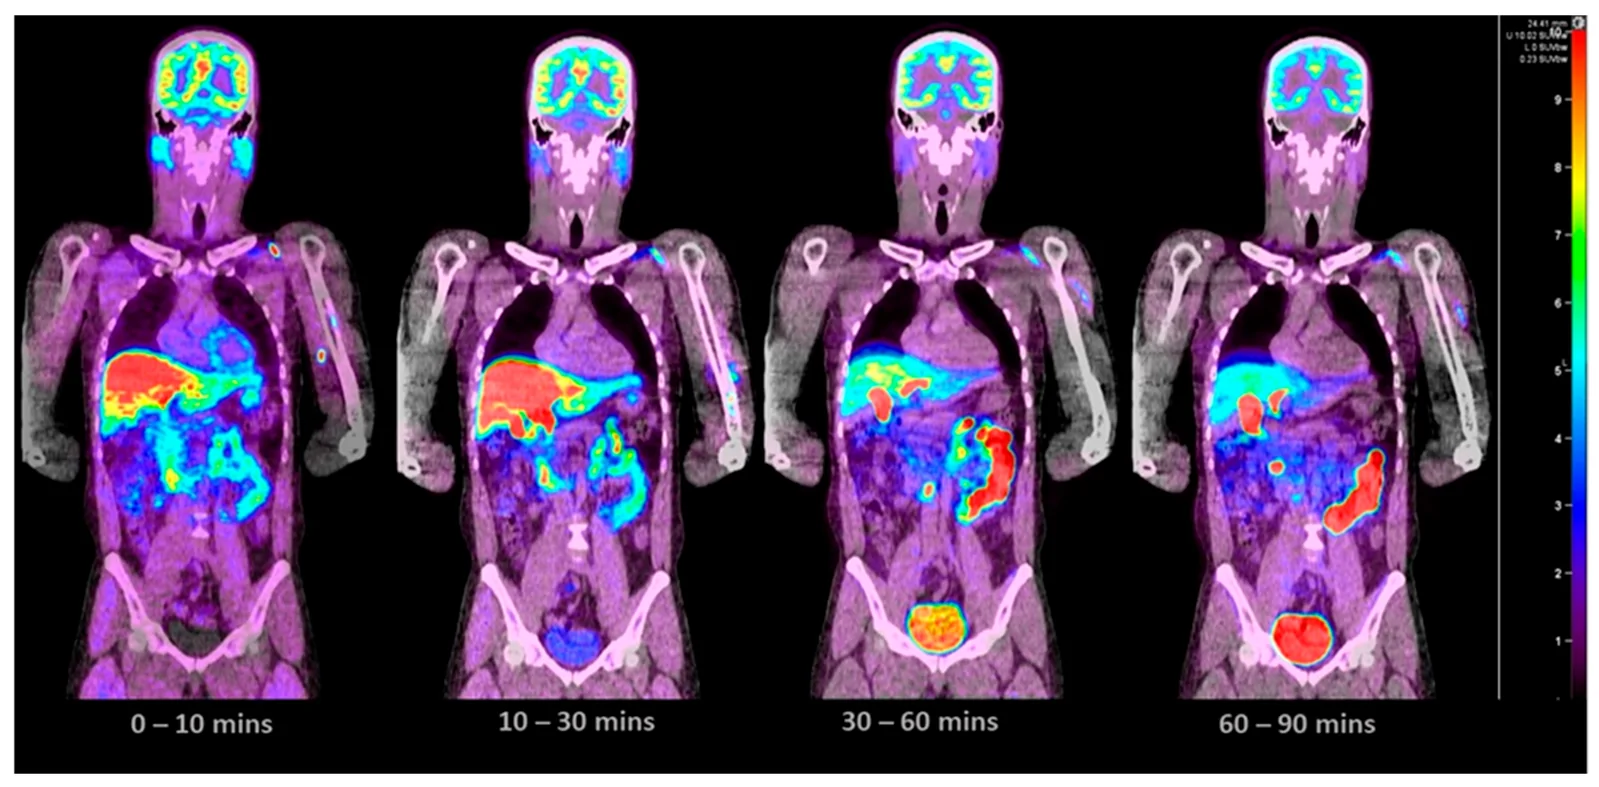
Sequential whole-body PET-CT images of a representative subject demonstrating biodistribution of [^18^F]FPEB. Rapid [^18^F]FPEB uptake is noted in the brain. Prompt [^18^F]FPEB tracer excretion is noted via both the hepatobiliary (as evidenced by tracer transit through the liver, followed by bowel) and the urinary system (as evidenced by urinary bladder tracer accumulation).
